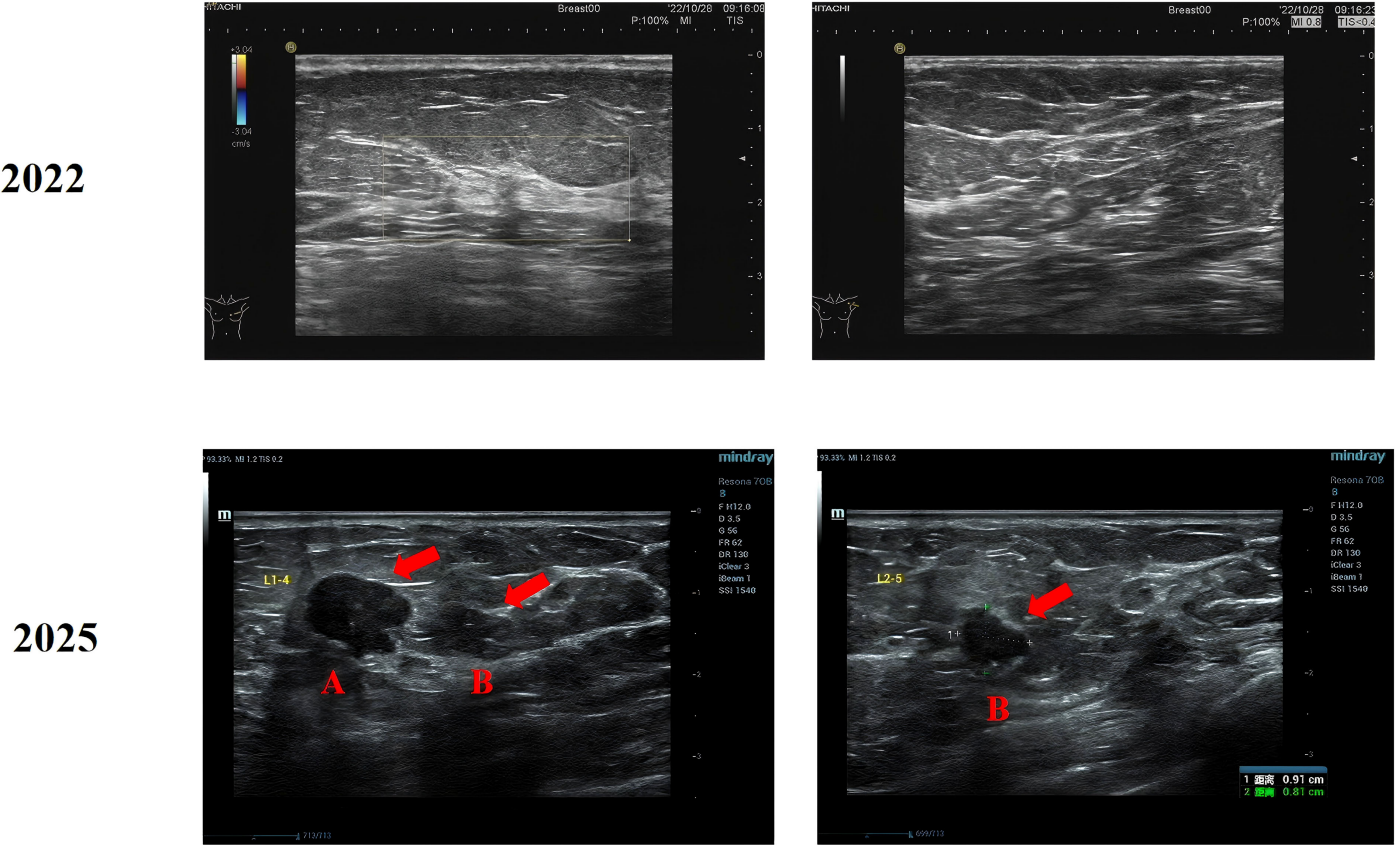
Comparative breast ultrasound images from 2022 and 2025. The 2022 examination revealed no definite space-occupying lesions. In 2025, two distinct masses were observed within the same imaging plane.
